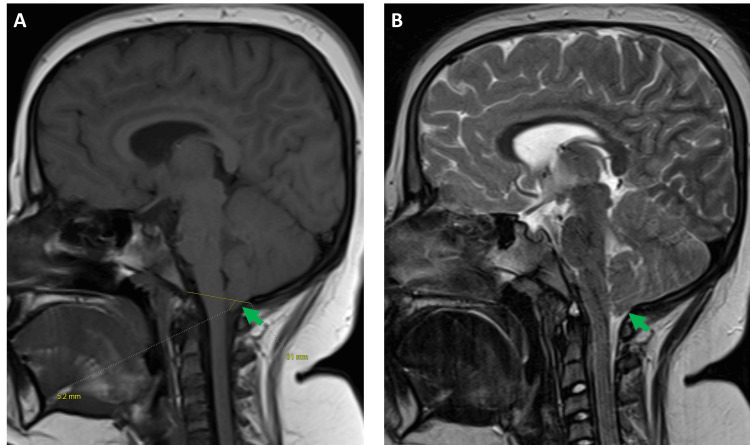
Magnetic resonance imaging (MRI) (A) Sagittal T1 and (B) sagittal T2 images demonstrate cerebral tonsils migrating 5.2 mm through the foramen magnum, causing indentation upon the cervicomedullary junction along with effacement of adjacent subarachnoid space (green arrow). No evidence of syringomyelia was found.
